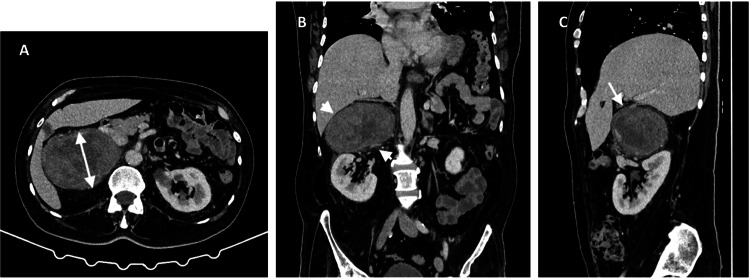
Infrahepatic mass in the region of the adrenal gland visible on the CT scan. The image shows an infrahepatic mass measuring 11.5 × 8.7 × 7.9 cm, indicated by arrows in a CT scan in axial (A), coronal (B), and sagittal (C) planes.
